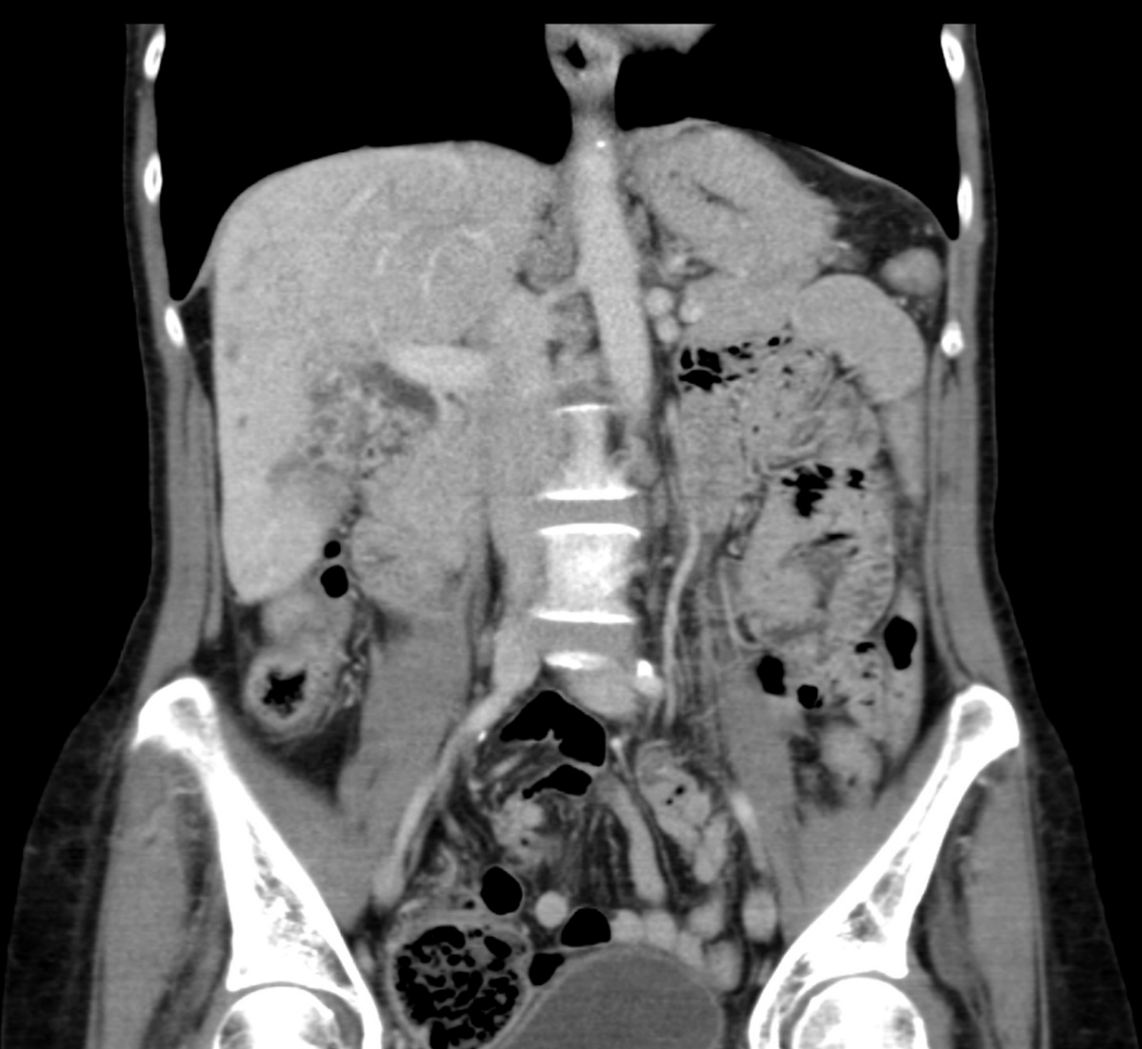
Representative coronal slice from CT obtained six months after second SIRT procedure
